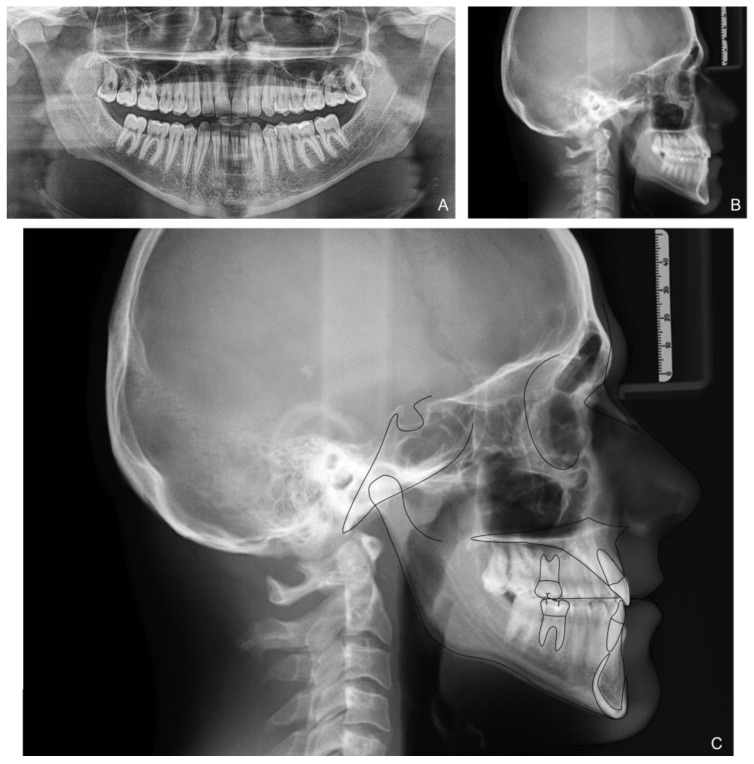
Patient X-rays and cephalometry after treatment: Orthopanoramic X-ray (A); Latero-lateral skull X-ray (B); Cephalometric tracing (C).
